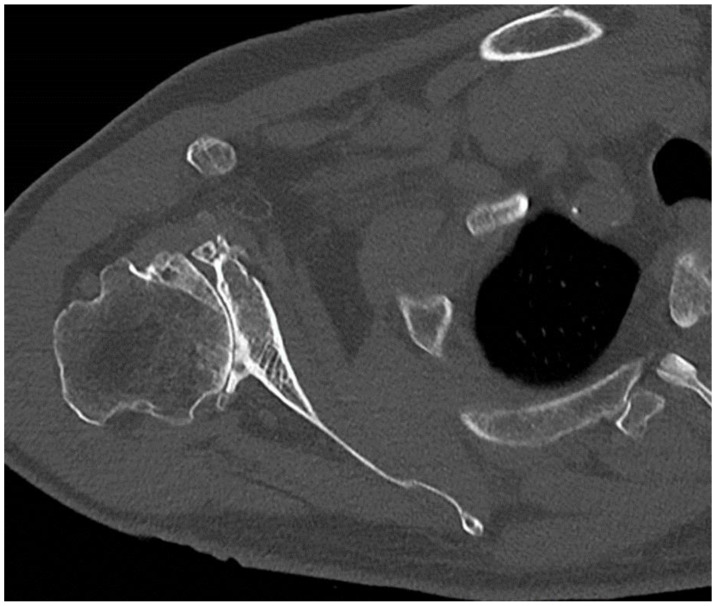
CT scan of the shoulder showing bone deformity due to osteoarthritis.
